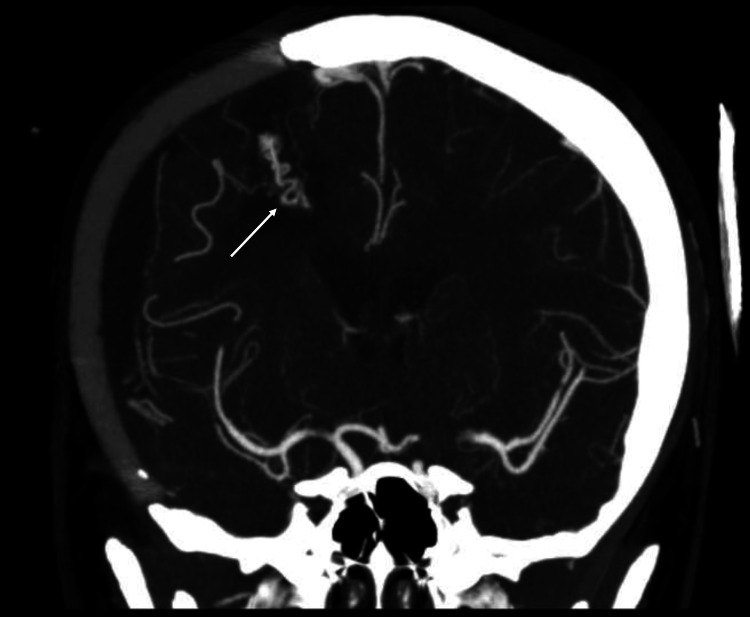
Routine computed tomography angiogram revealing recurrence of the brain arteriovenous malformation (solid arrow) in the same location as the one excised three years ago
